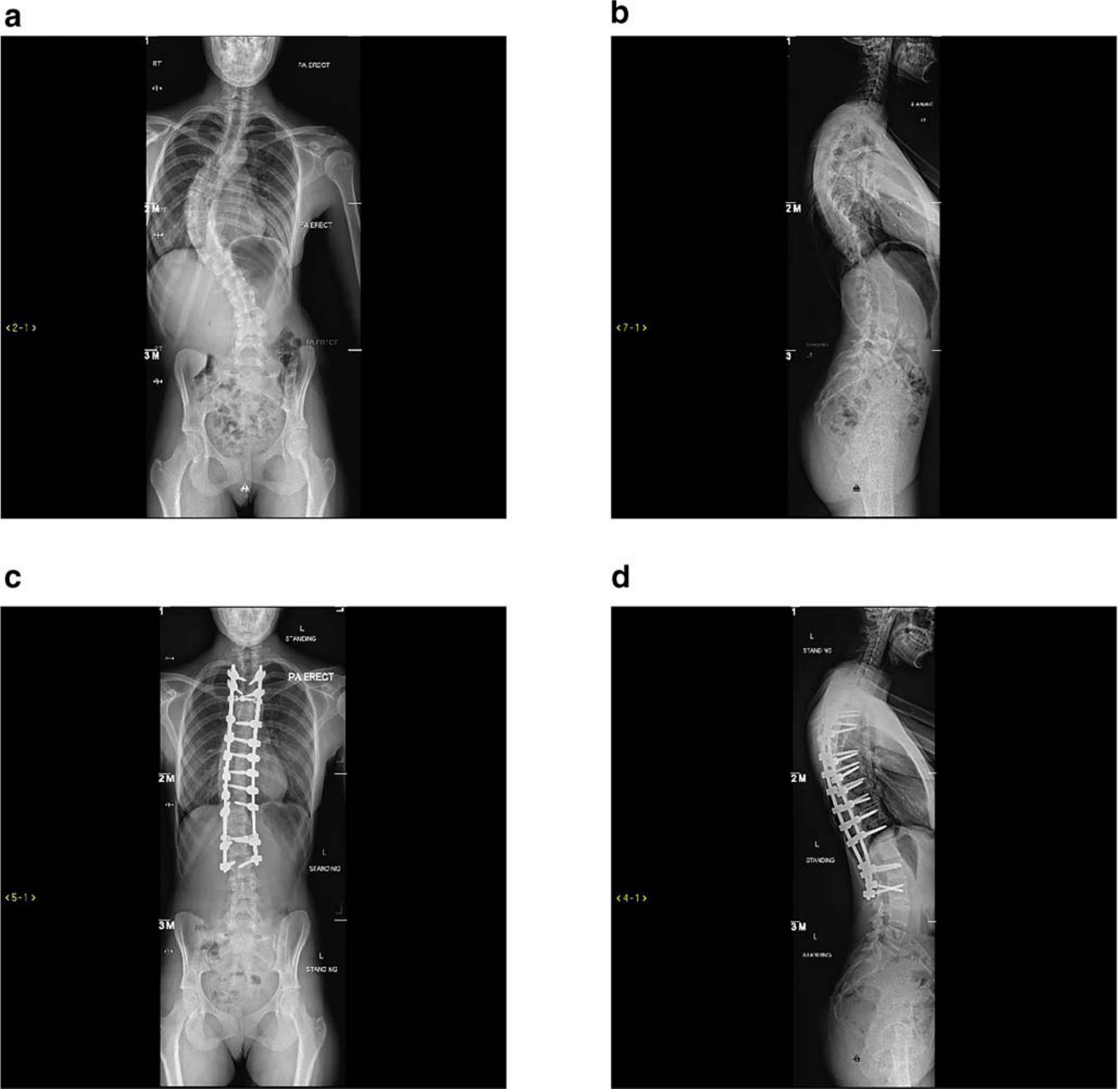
Posterior anterior and lateral preoperative radiographs of a female patient with adolescent idiopathic scoliosis (a, b) and postoperative radiographs after posterior surgical approach (c, d)
